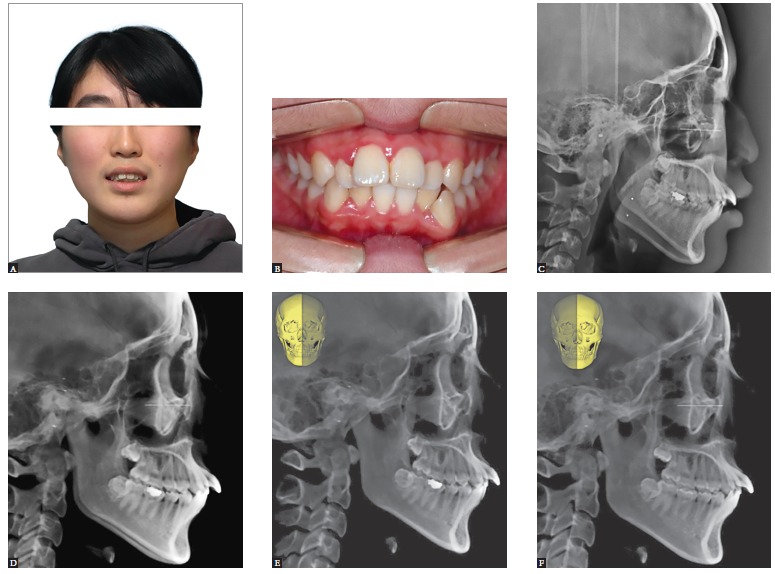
Generation of cephalograms from cone-beam volume image. A 20-year-old
